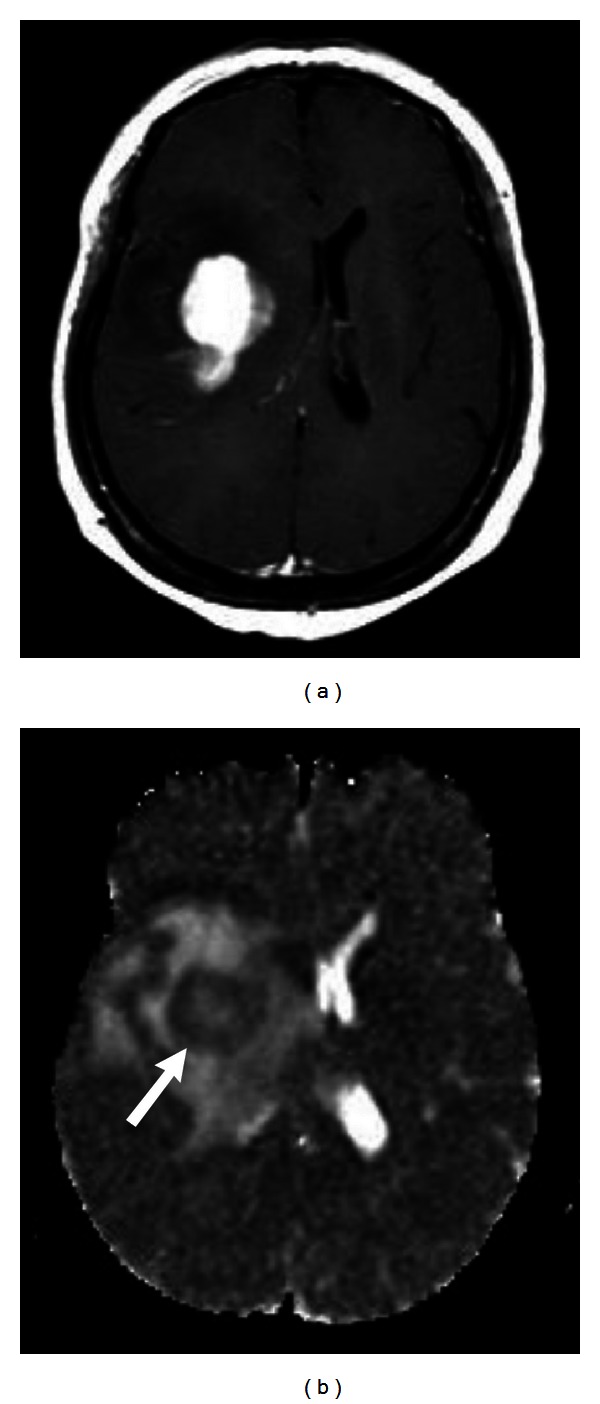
(a) Contrast-enhanced T1-weighted image shows a glioblastoma with strong enhancement after intravenous gadolinium injection. (b) The tumor shows decreased ADC values on ADC map (arrow in (b)).
